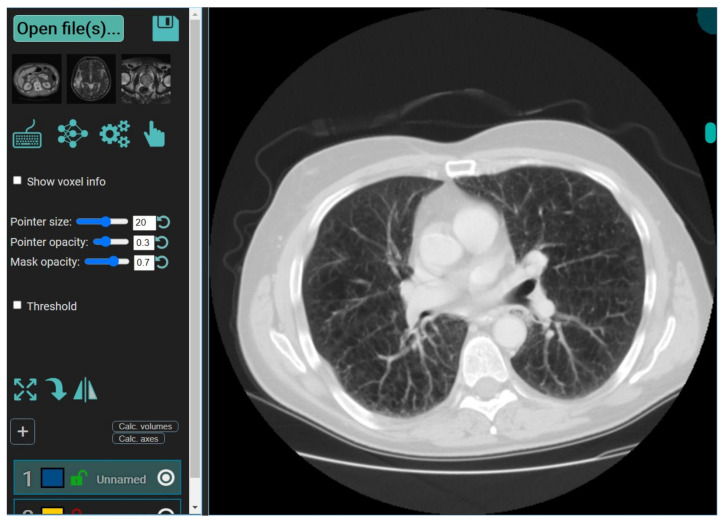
Display of CT image using the MedSeg tool.
